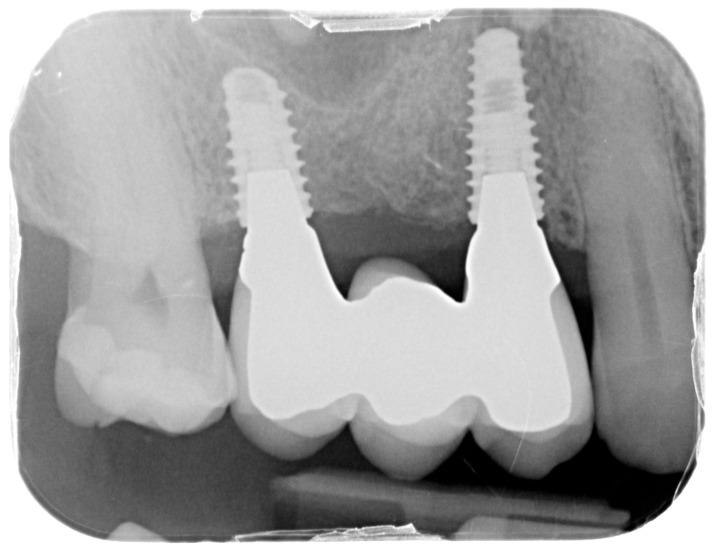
Intraoral X-ray at one year follow-up.
